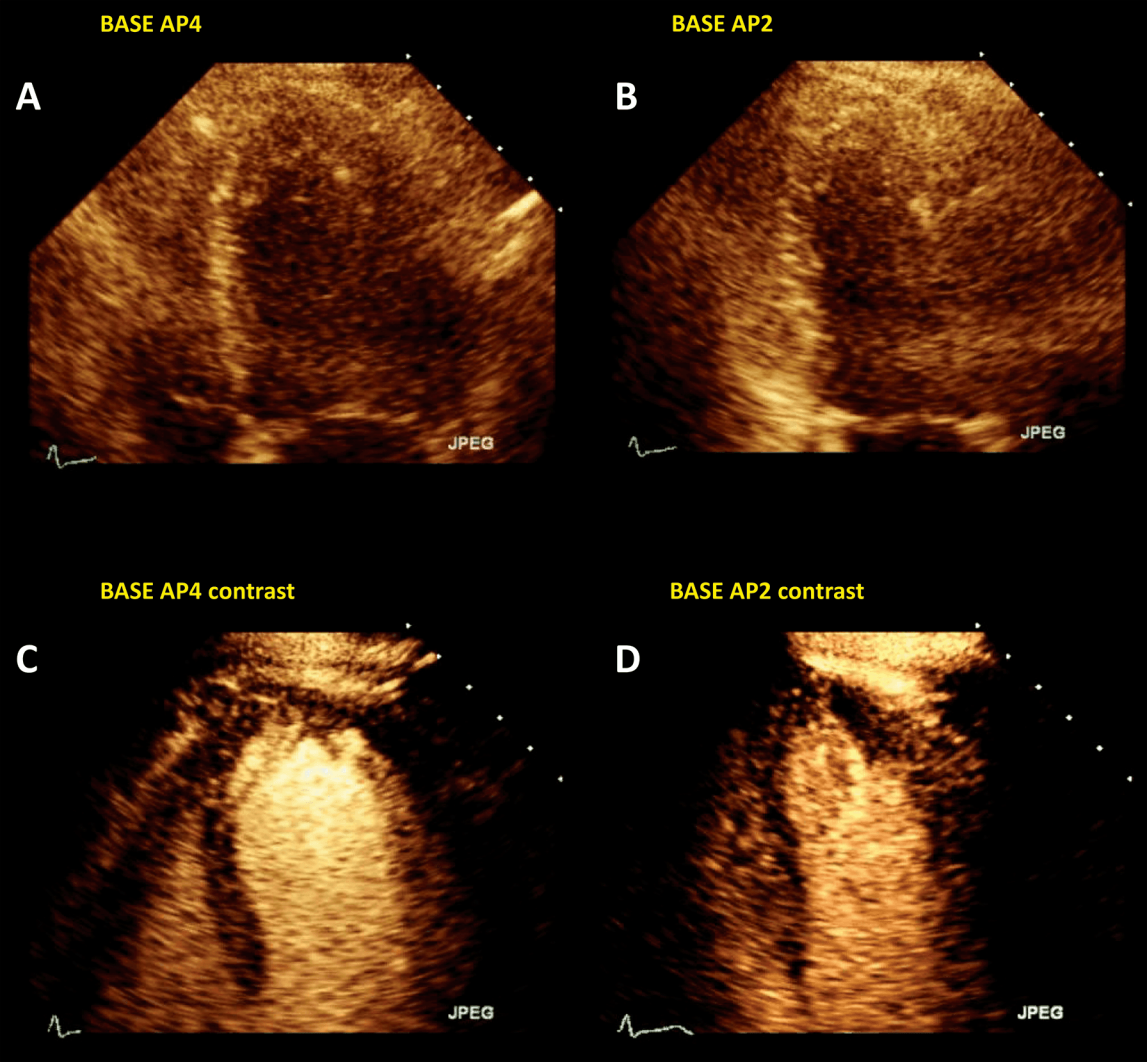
Microbubble enhanced stress echocardiography. Baseline
